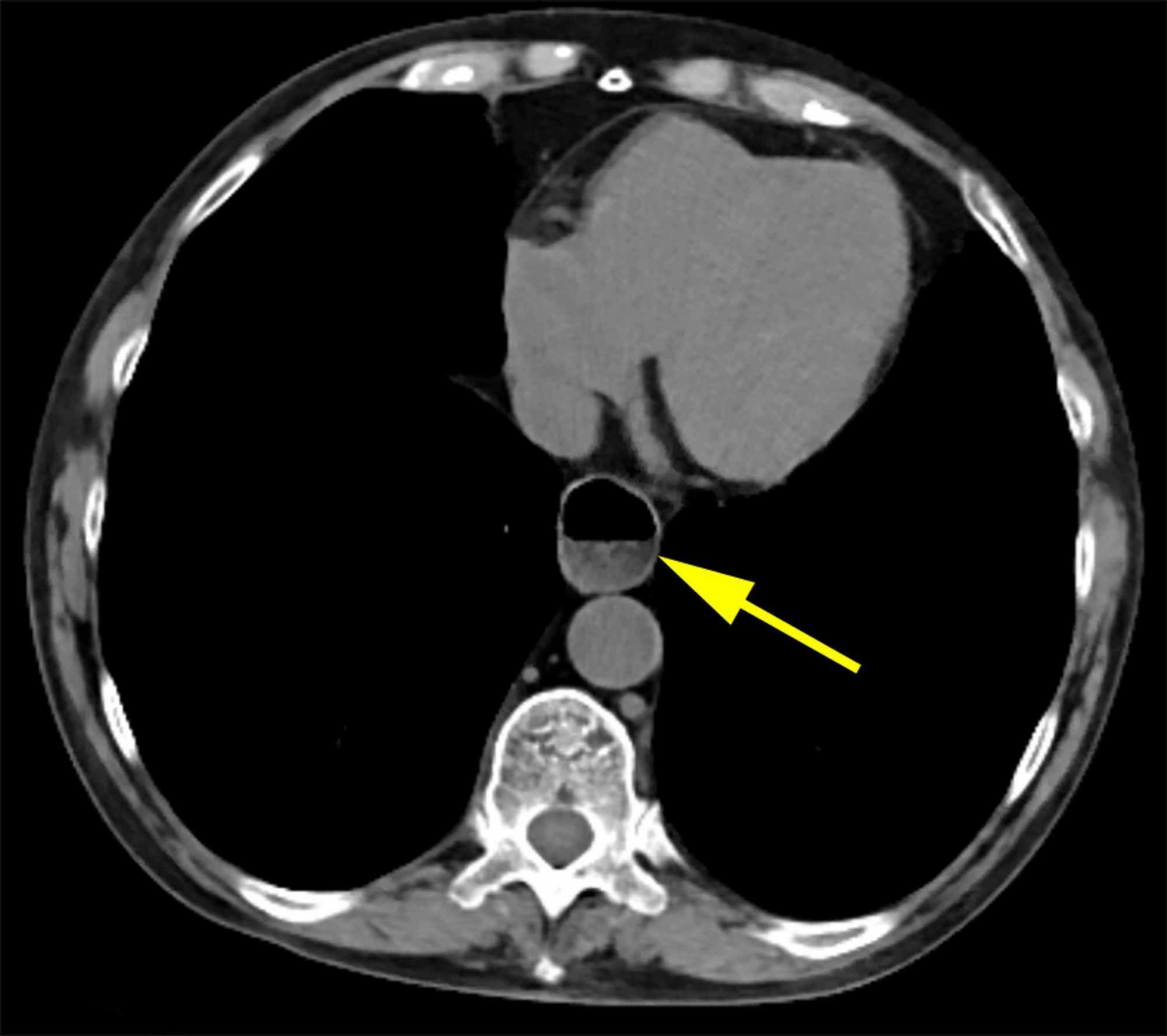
CT scan demonstrating a fluid-filled dilated esophagus
